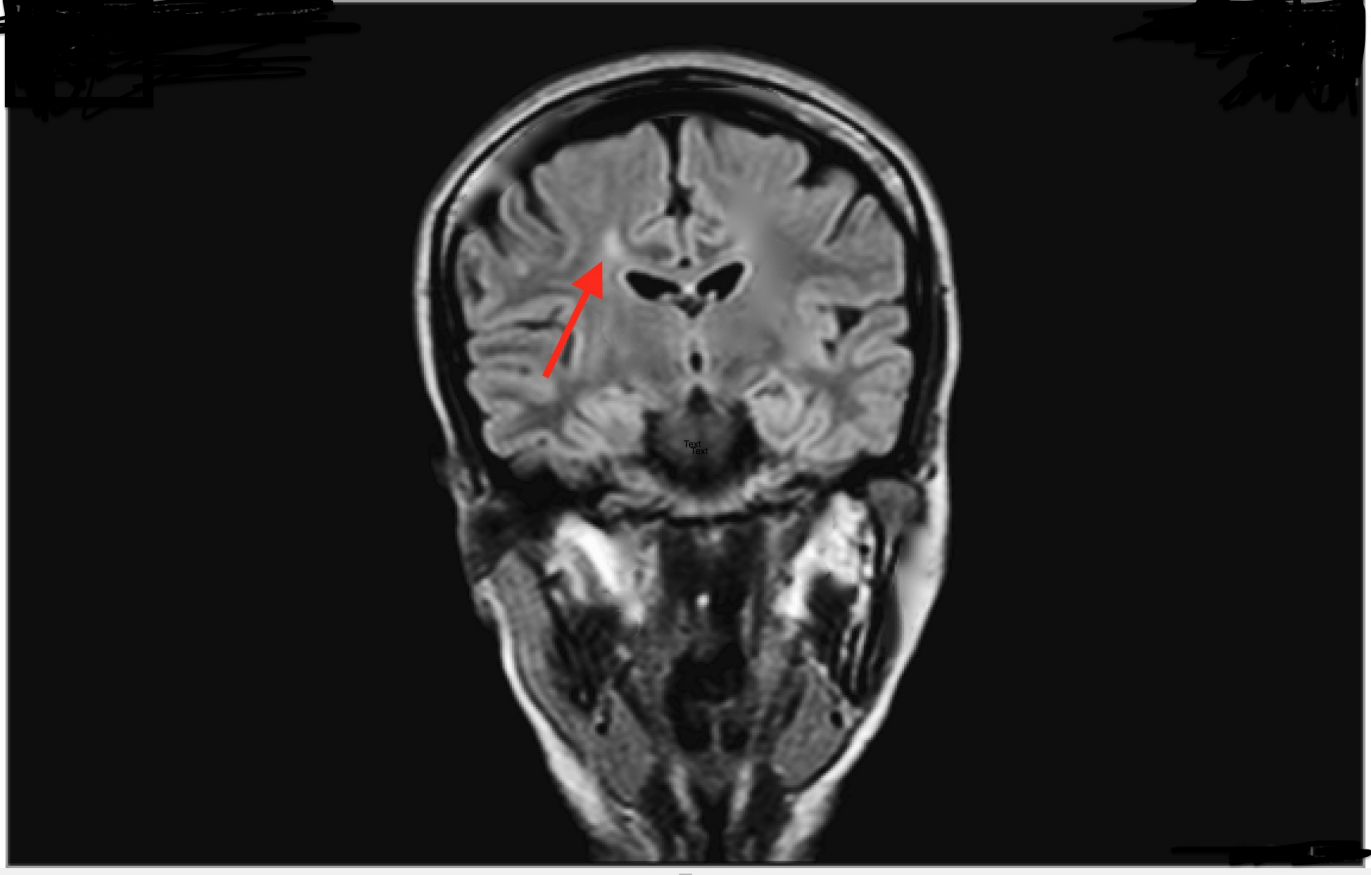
Hyperintense lesions at T2 weighted image (T2WI) and fluid-attenuated inversion recovery (FLAIR) sequence shown by arrow.
